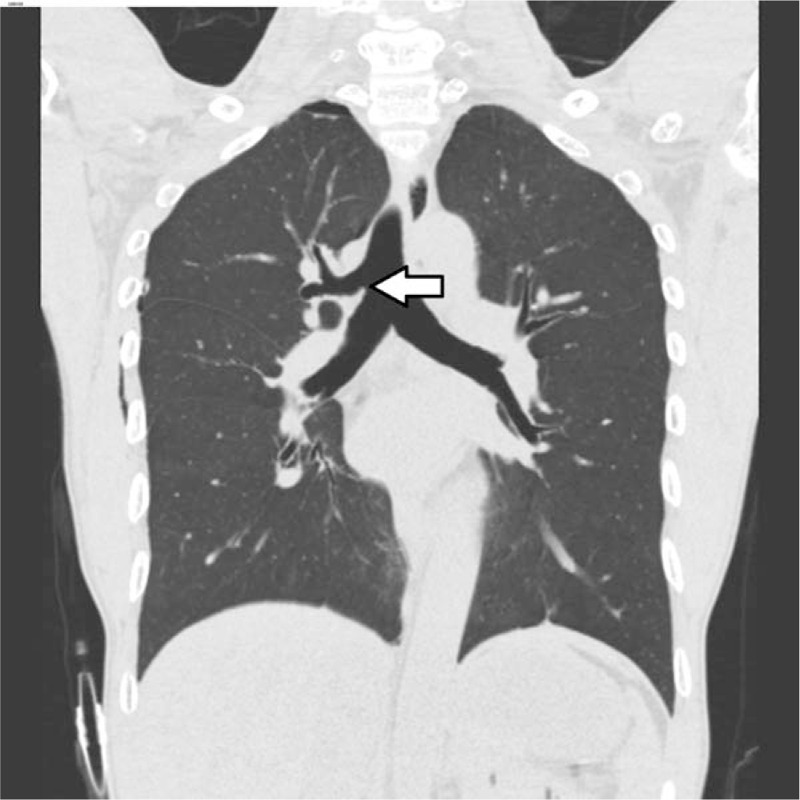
Preoperative chest computed tomography demonstrates a tracheal bronchus supplying the right upper lobe and originating from the right lateral tracheal wall, 1.8 cm proximal to the carina on the coronal reconstructed image (arrow).
